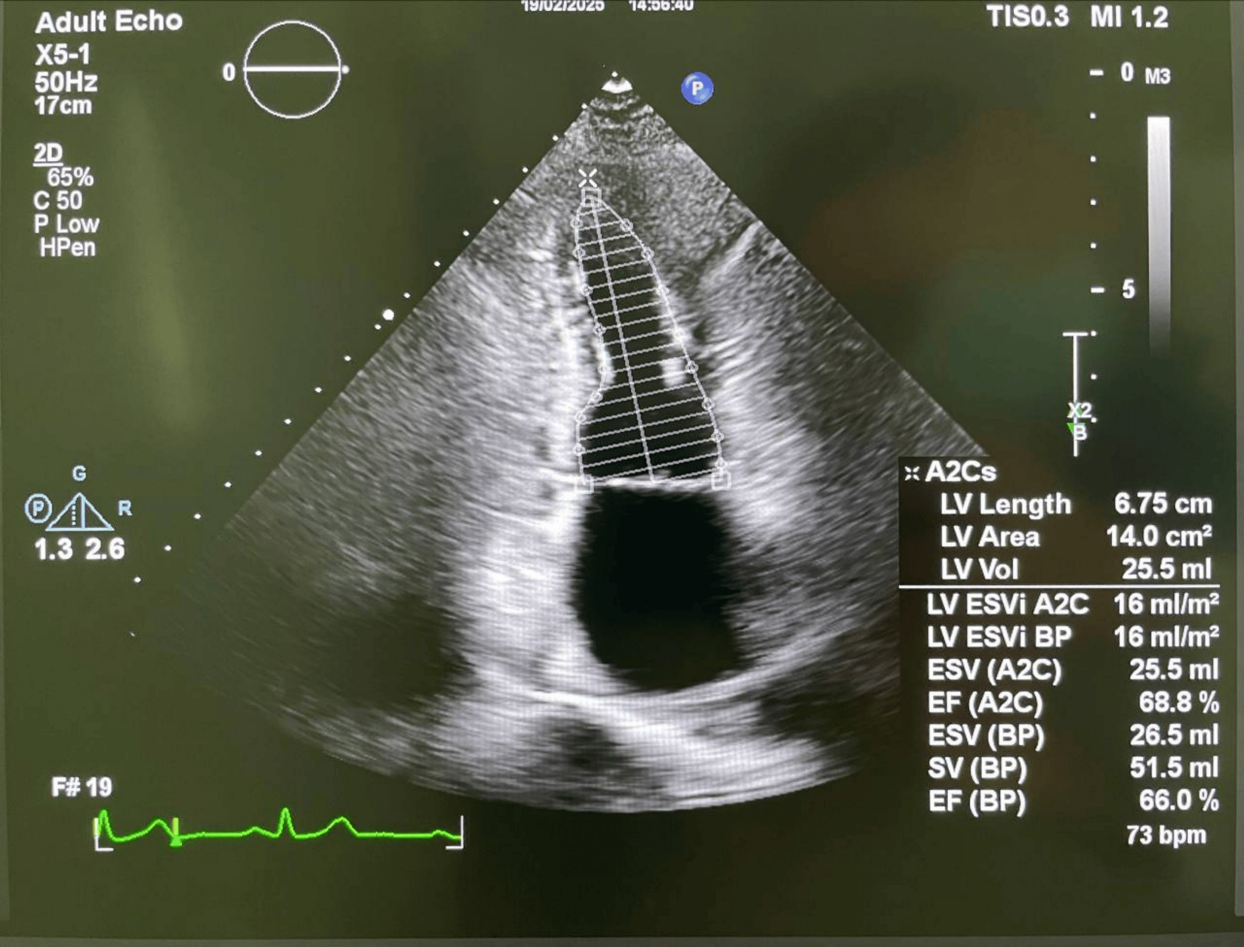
Apical two-chamber echocardiographic view demonstrating left ventricular systolic function. The image shows the endocardial borders traced for biplane Simpson method calculation, with a left ventricular ejection fraction of 66%. Mild concentric remodelling and preserved systolic function are noted.
